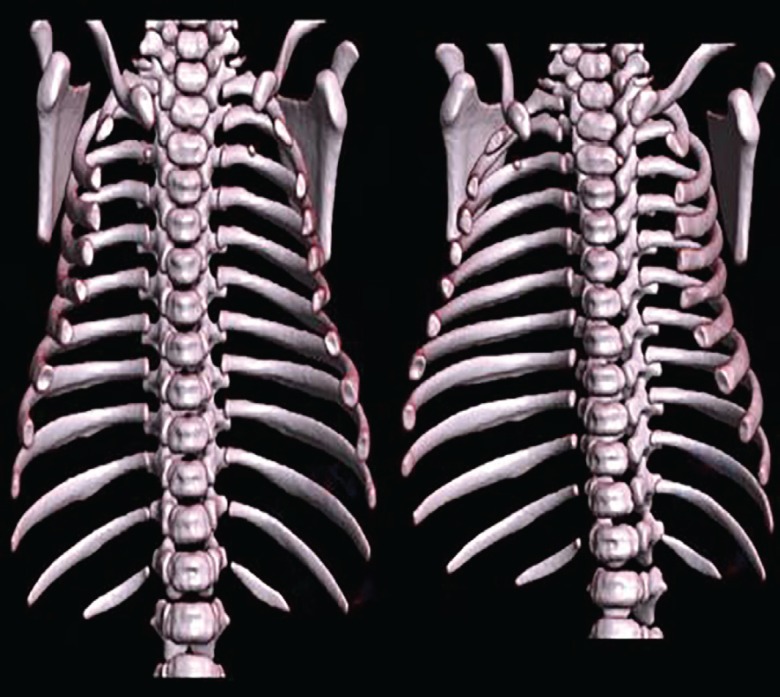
Three dimensional reconstruction of CT scan of chest
